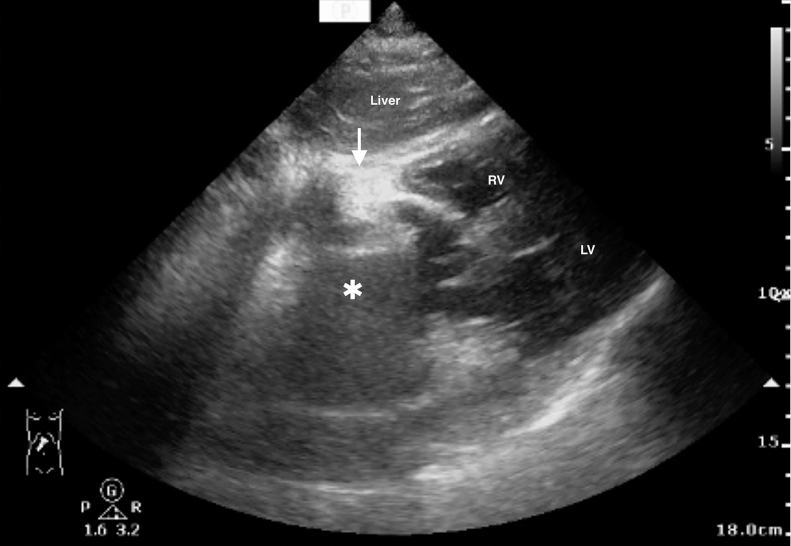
Point-of-care ultrasonography with subcostal four-chamber view demonstrates the liver (superior), left ventricle (LV), and right ventricle (RV). Multiple hyperechoic foci (arrow) with posterior dirty shadowing artifacts (asterisk) fill the pericardial space, consistent with pneumopericardium. The right atrium is completely obscured by the air artifacts.
